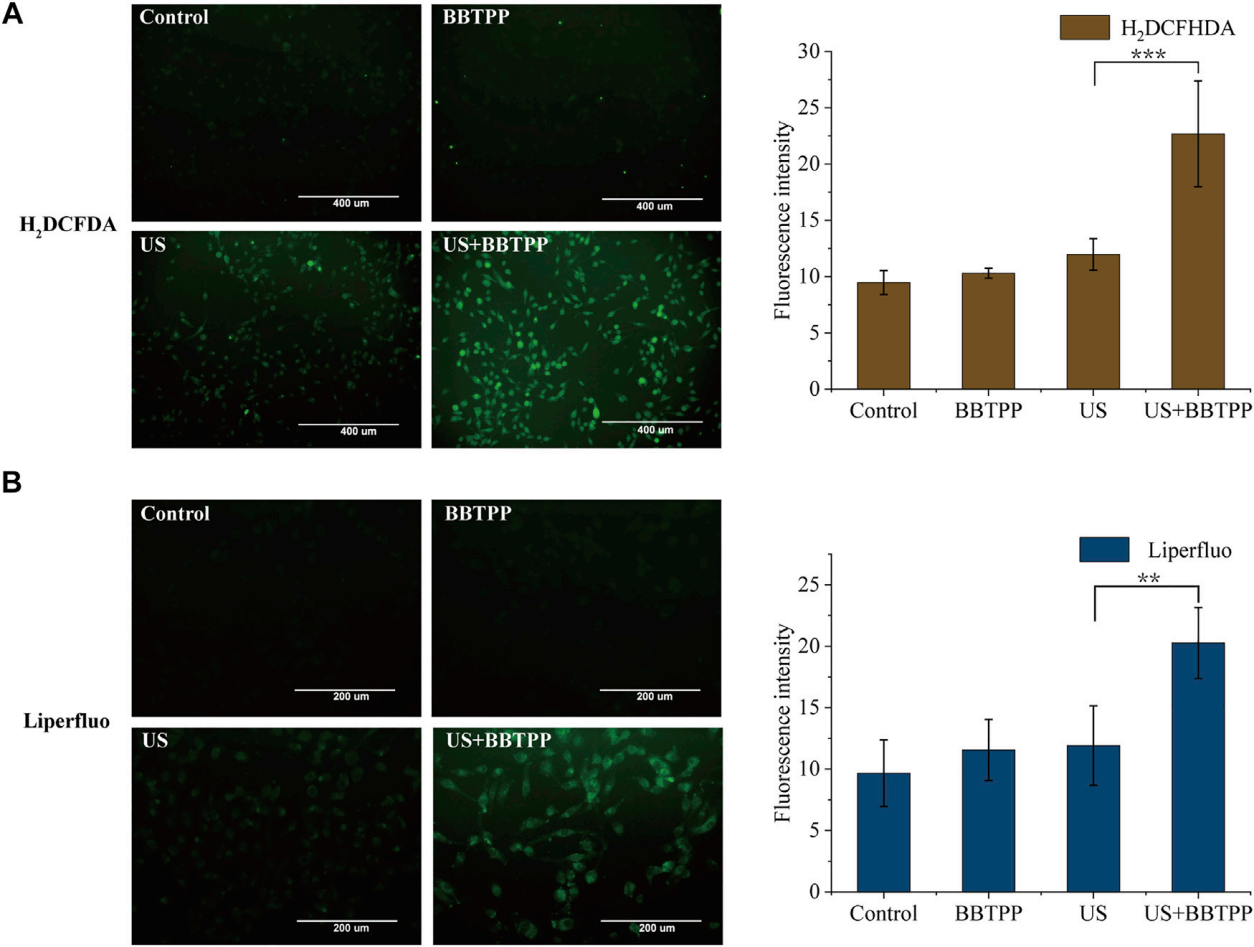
Intracellular ROS production and lipid peroxidation of PC-9 cells under different treatments (A) Representative images of intracellular ROS production measured by H_2_DCFDA and the fluorescence intensity of H_2_DCFDA analyzed by ImageJ (B) Representative images of lipid peroxidation measured by Liperfluo and the fluorescence intensity analyzed by ImageJ. The data were presented as the mean ± standard deviation. US: ultrasound; *p*<0.01, p*<0.001.
